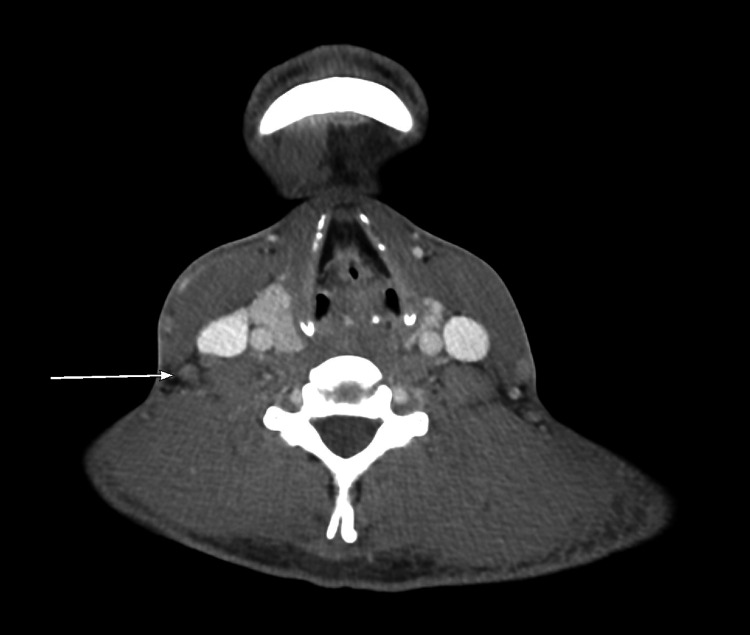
CT scan neck (axial view) at the time of admission View of right-side borderline-enlarged lower cervical and supraclavicular lymph nodes.
